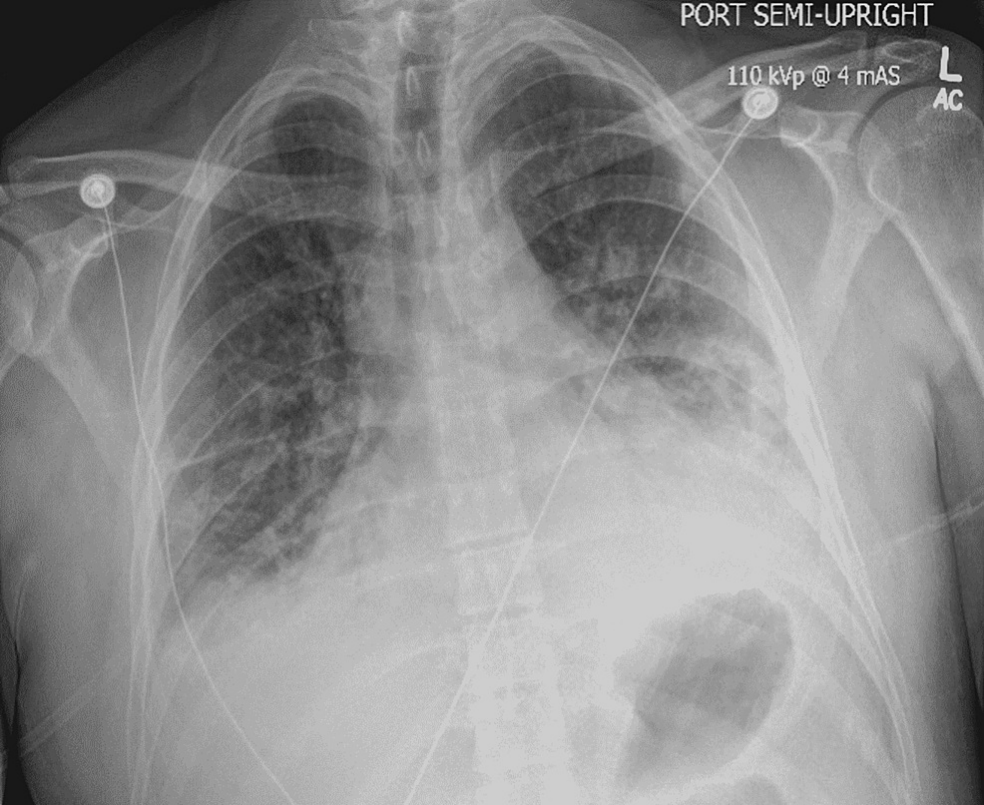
Chest X-ray demonstrating pulmonary edema and bilateral pleural effusions.
